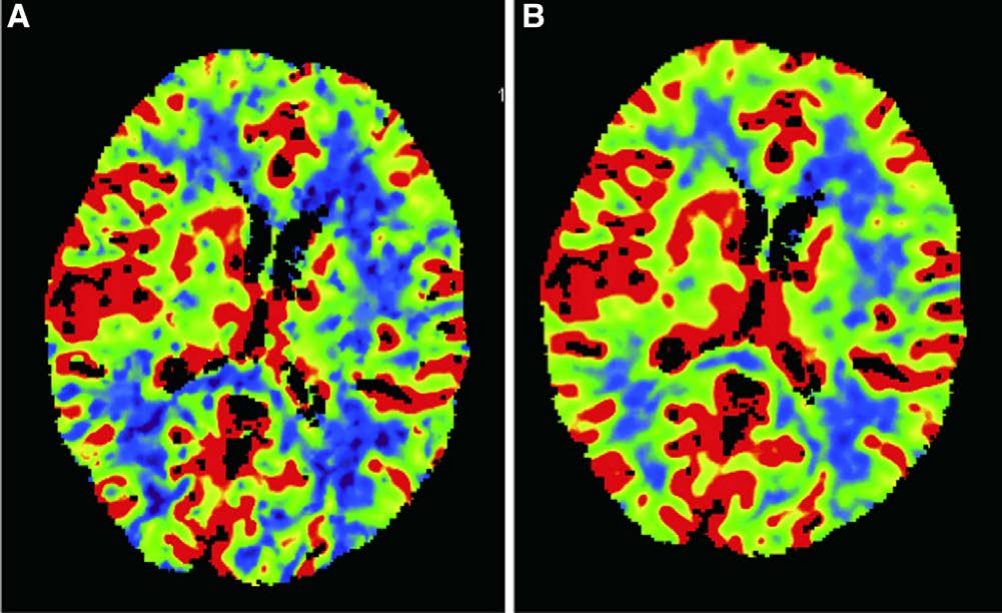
Computed tomography perfusion (CTP) showed hyperperfusion in the right basal ganglia.
